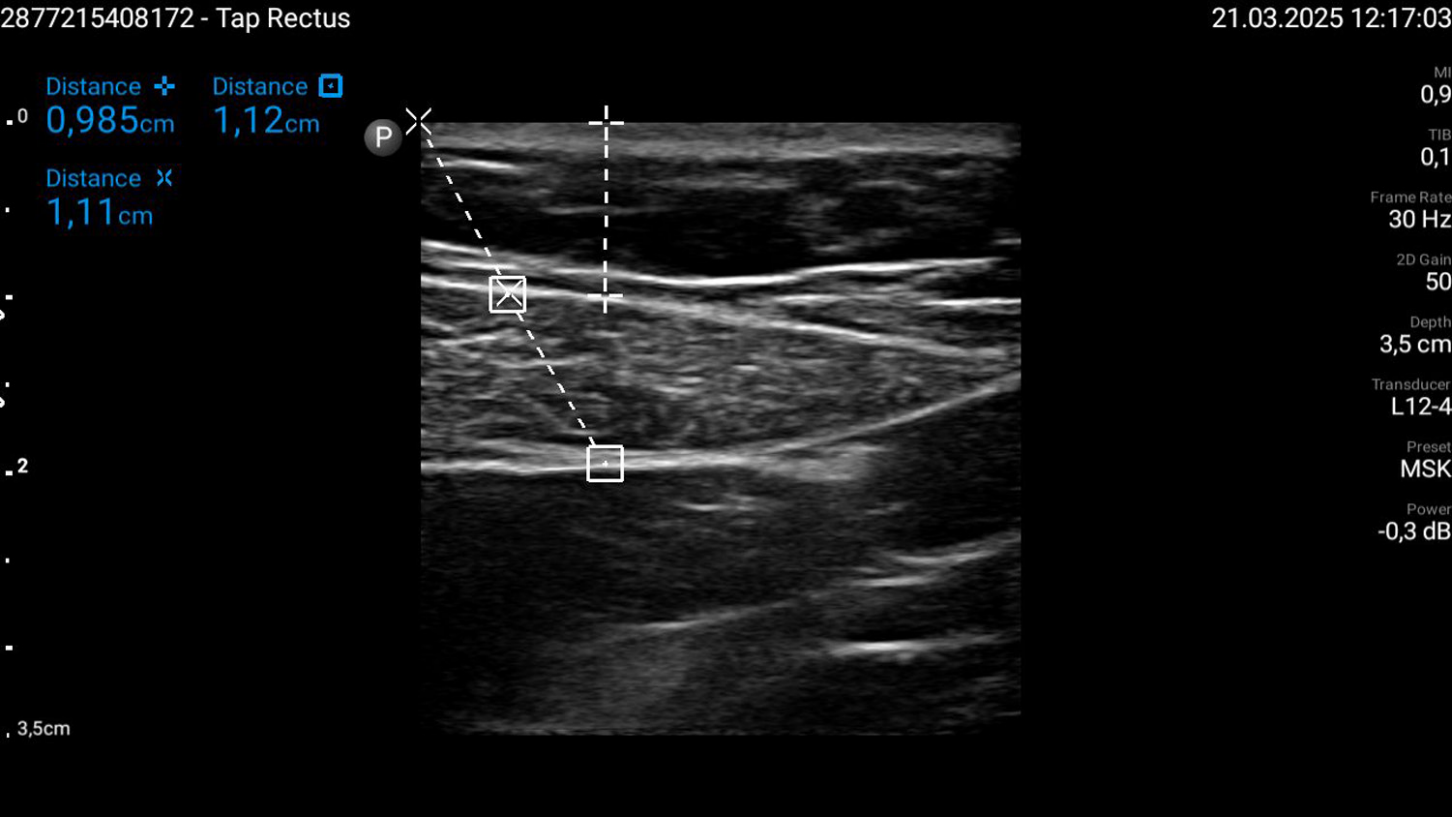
Ultrasound scan of the region of interest for performing a rectus sheath block in a normal weight patient obese patient. The ‘Cross’ represents the perpendicular distance from the skin to the rectus abdominis muscle, the ‘X’ indicates the hypothetical needle path from the skin to the rectus abdominis muscle, and the ‘Square’ denotes the rectus abdominis muscle.
